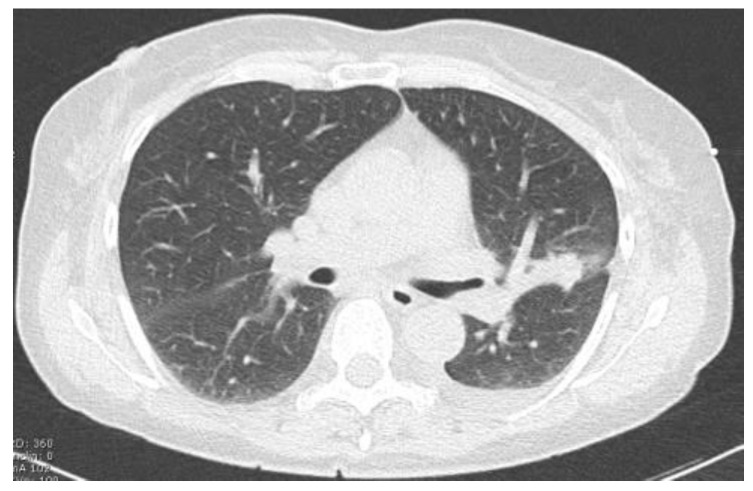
CT follow up after radiopharmaceutical injection.
